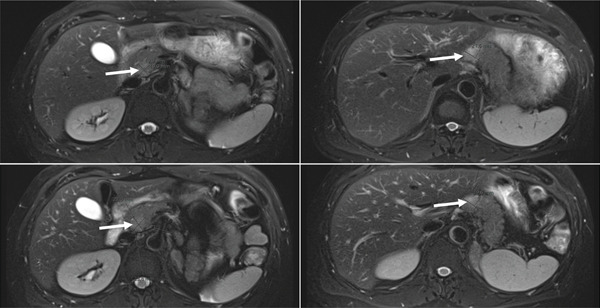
Axial abdominal MRI findings consistent with pancreatic metastases (white arrow).
